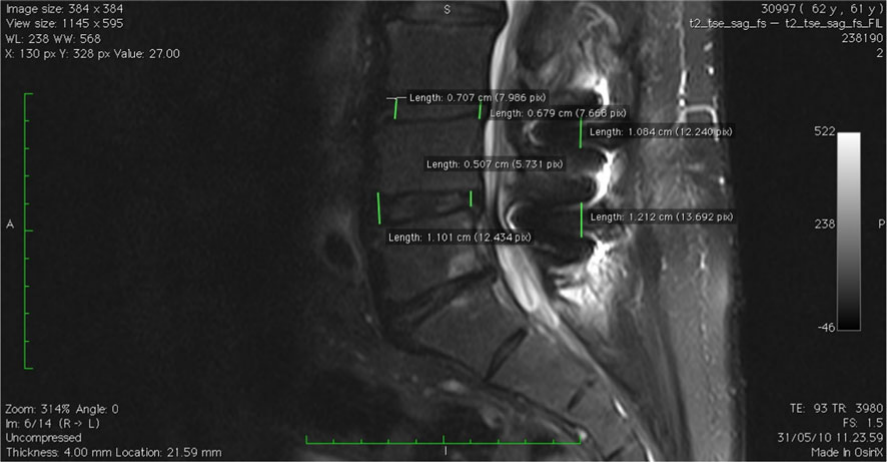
MRI postoperative measurement
